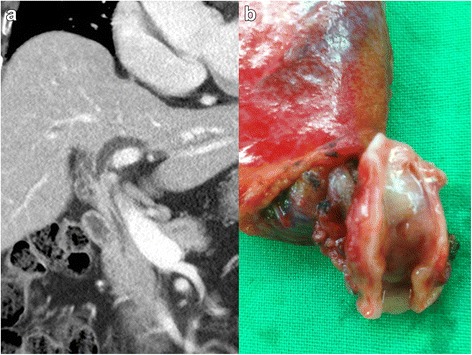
Preoperative computed tomographic (a) and gross (b) findings. a Diffuse dilatation of the intrahepatic duct and wall thickening of the common bile duct were shown. b Wall thickening with focal mass was found without complete obstruction by mass
